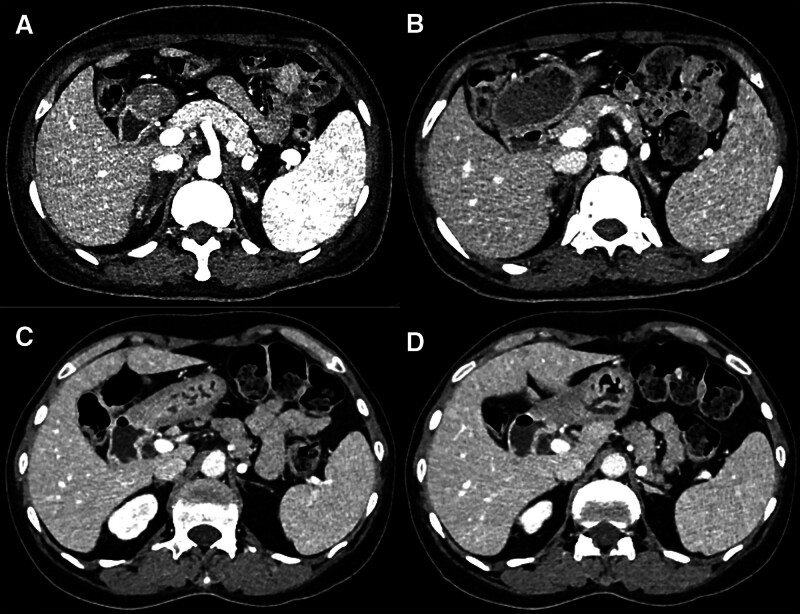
The changes of adrenal infarction in the subsequent CT reviews. (A and B) The first and second CT reviews show the adrenal glands atrophy gradually. (C and D) The last CT review after 1 yr shows the obviously absorbed of lesion, with a scanty amount of adrenal gland remnant. CT = computed tomography.
